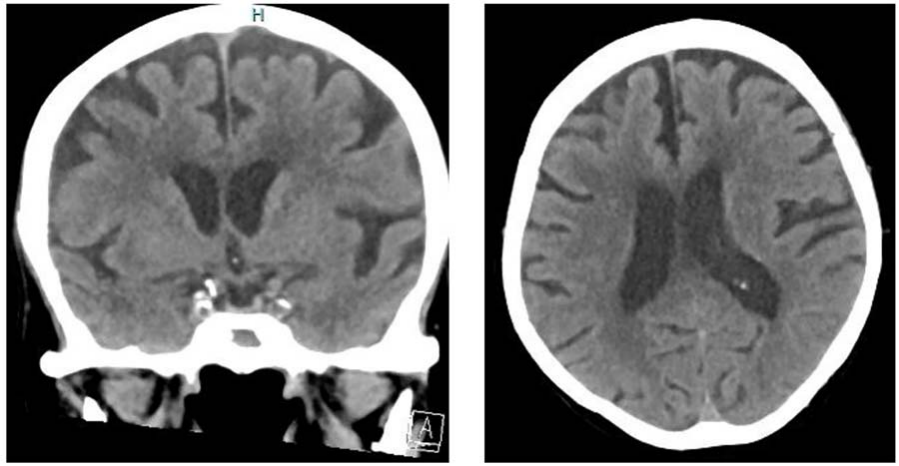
Computed tomography of the head demonstrated diffuse parenchymal volume loss and chronic microvascular changes but no acute intracranial pathology.
